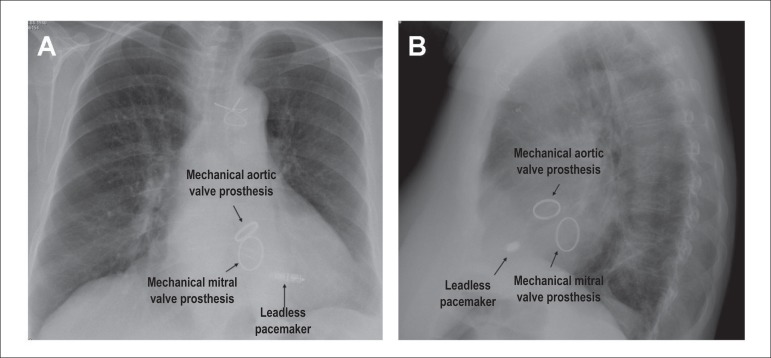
Chest X-Ray of the patient (panel A, posteroanterior view; panel B:
